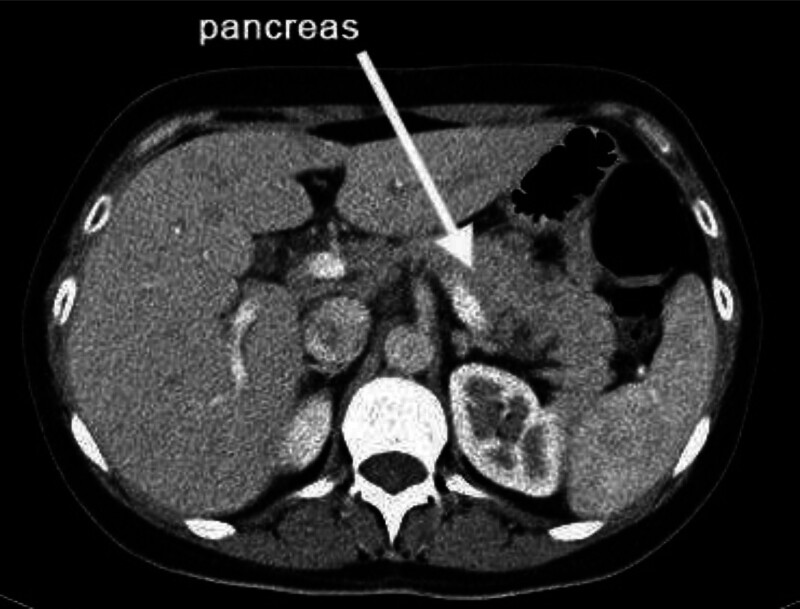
CT scan showed a normal pancreas (arrow). CT = computer tomography.
